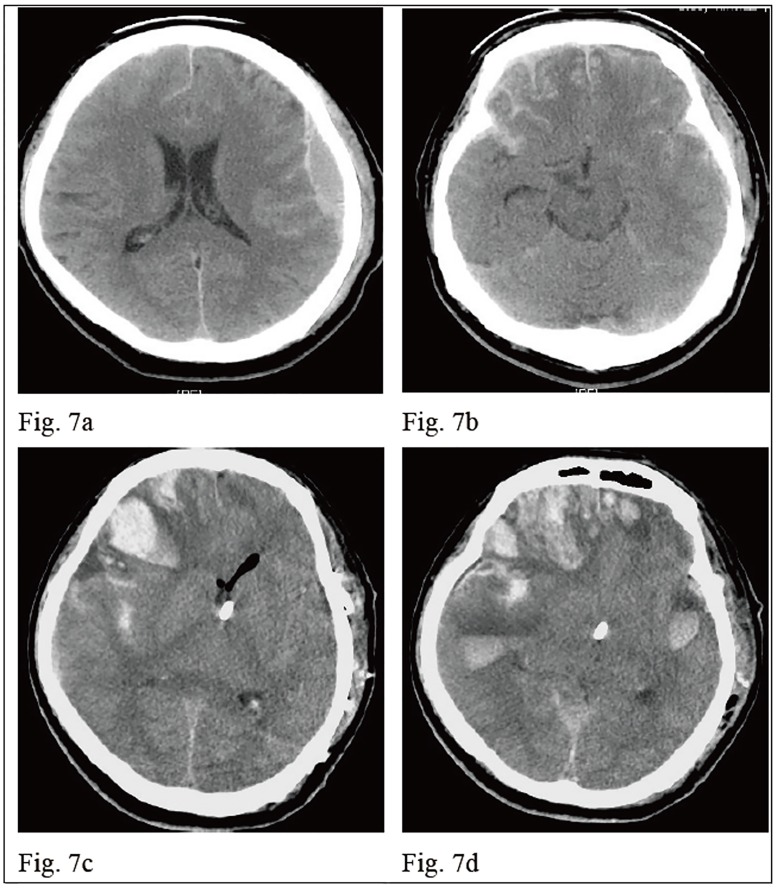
The progression of brain CT scans in a traumatic brain injury patient (case 3). Fig 7a. and 7b show that the initial brain CT revealed left fronto-temporal EDH and bilateral frontal and temporal small contusion hemorrhages, and SAH. Fig 7c. and 7d show that the follow-up brain CT after left craniotomy on POD 1 revealed right frontal and temporal contusion hemorrhage progression and right SDH with a midline shift to the left, left frontal and temporal contusion hemorrhages, pneumocephalus, diffuse brain swelling and bilateral slender ventricles. The EVD tube was placed in the left frontal horn.
